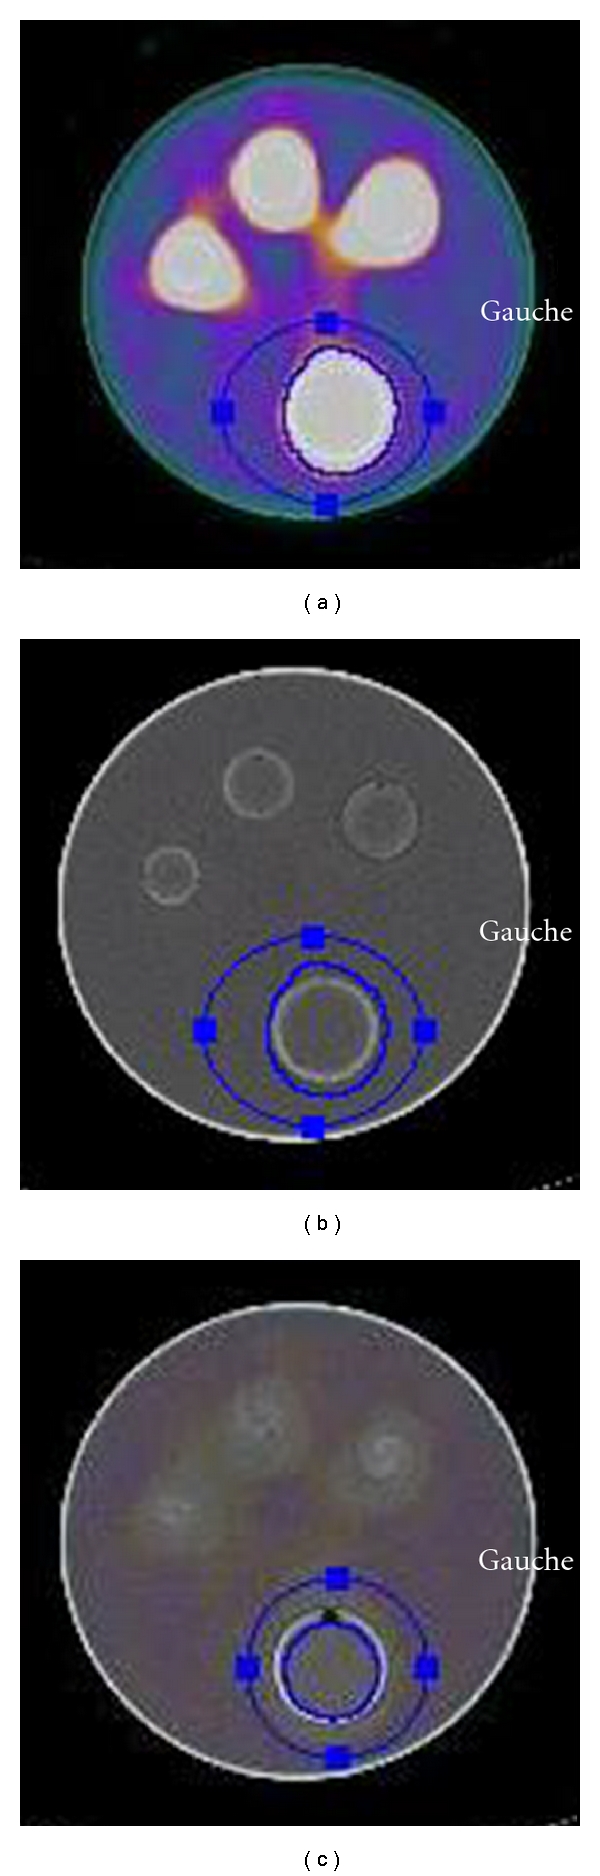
Delineation of VOIs used for quantitative analysis of SPECT and SPECT/CT analysis (a, b): VOI defined on SPECT hot spot alone. Fused SPECT/CT image with the VOI matching with the hot spot ((a), SPECT/CT scale); CT scale showing that the VOI is not accurate and larger than the sphere (b). (c): VOI defined with SPECT/CT and using the CT scale: the isocontour was fitted by superposition on the boundaries of the internal wall of the sphere.
